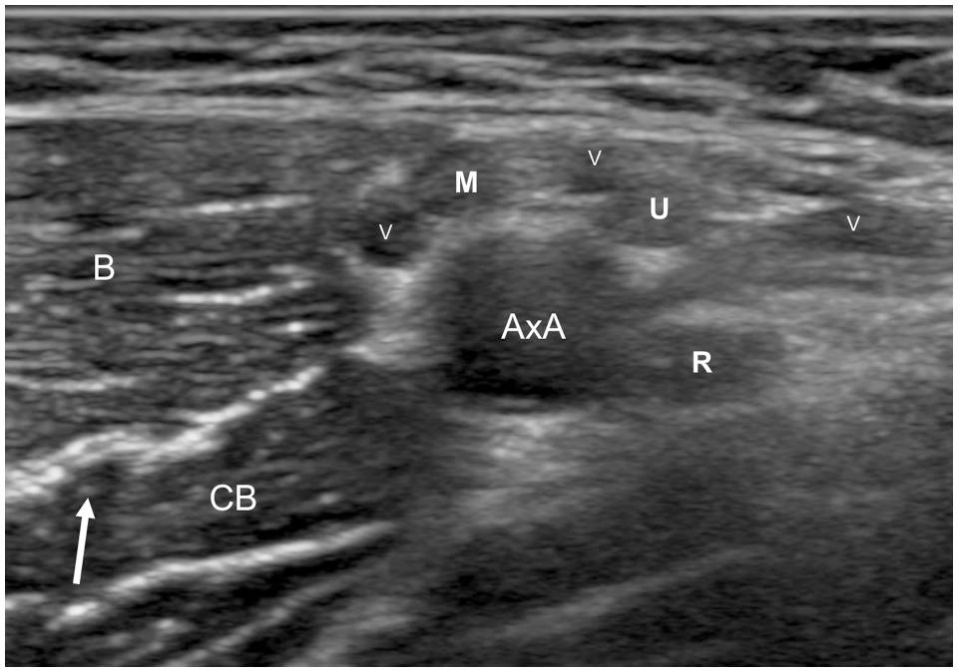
Transverse ultrasound of axillary fossa showing the musculocutaneous nerve (MCN) lying between the biceps (B) and coracobrachialis (Cb) muscles. The median nerve (M) consistently lies closest to the biceps muscle. The radial nerve (R) tends to lie towards the posterior of the axillary artery while the ulnar nerve lies superomedial to the axillary artery between the radial and medial nerves.
